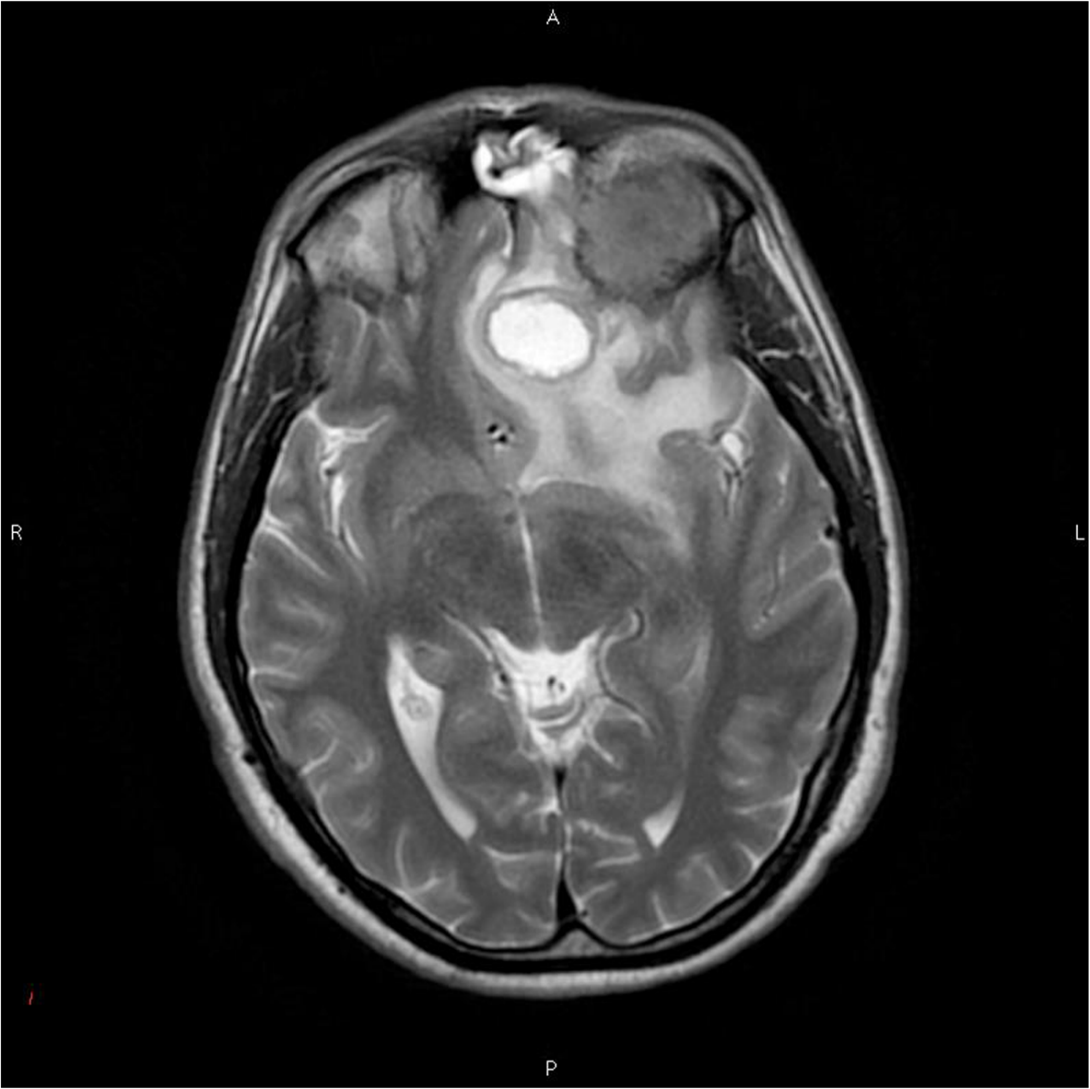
Axial T2 MRI showing a round hyperintense cystic area with surrounding vasogenic edema, and orbital involvement along with left-to-right midline shift due to mass effect
